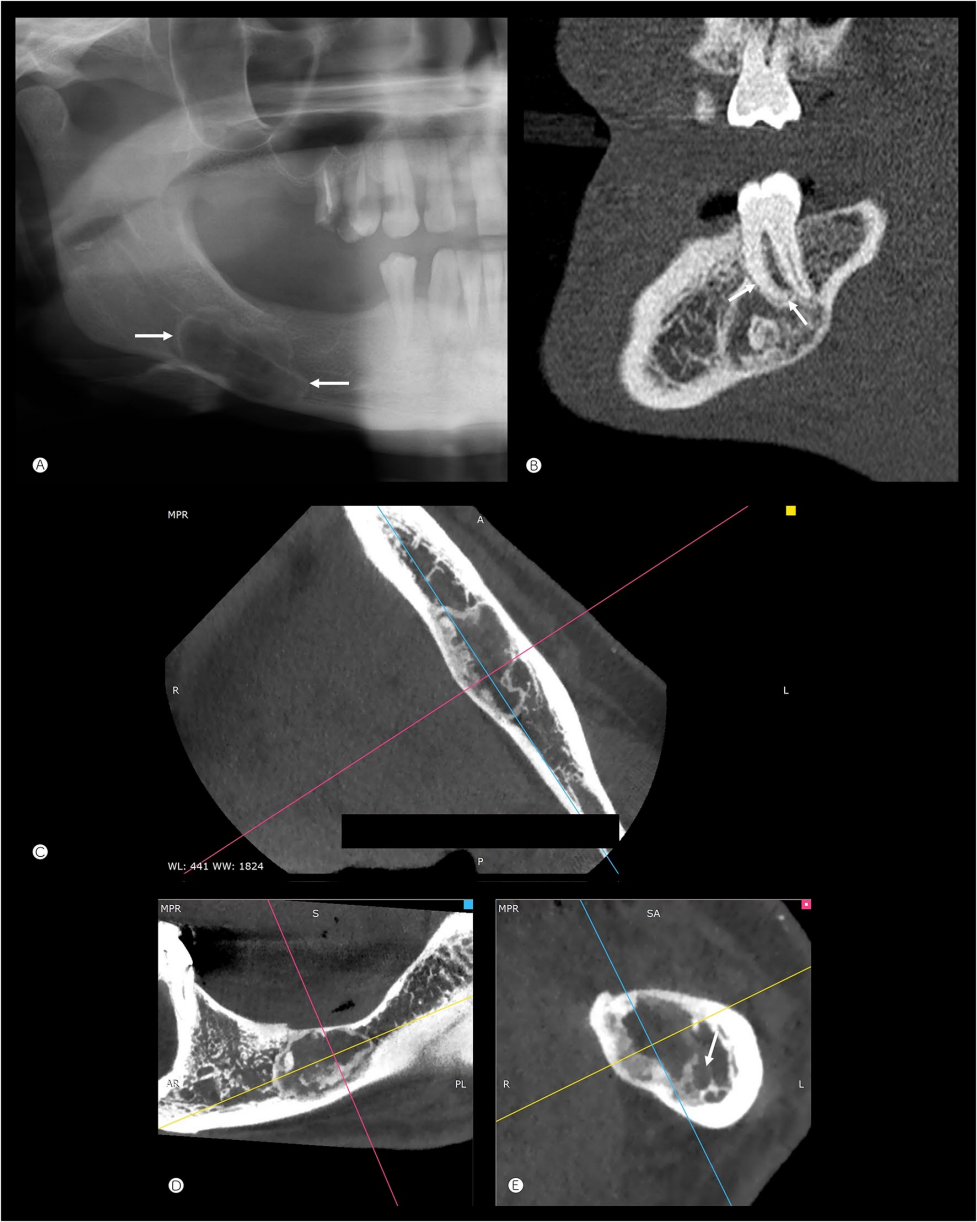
Relationship to tooth-bearing areas. A A lesion with a thin sclerotic margin (arrows) is predominantly inferior to the mandibular canal, scalloping the adjacent inferior cortex of the bone (case 20). B Sagittal CT reconstruction of the left mandible (case 16) showing remodeling of the tooth root but an intact periodontal ligament space (arrows) and lamina dura. C–E Multiplanar reconstructed CT of a lesion in the body of the left mandible (case 15) involving the edentulous tooth-bearing region. The mandibular canal is located inferolateral to the tumor (arrow in bottom right (oblique coronal) image)
